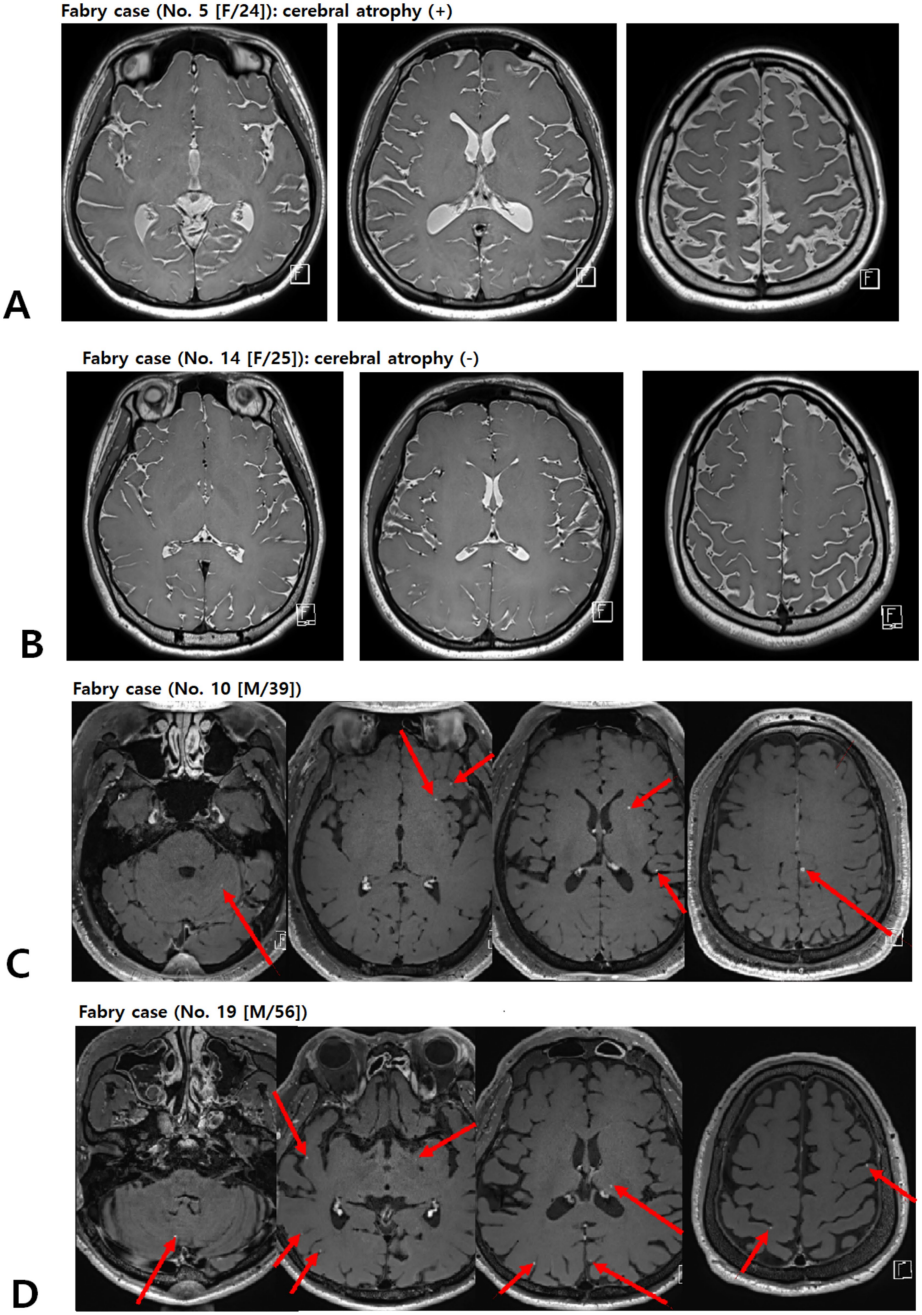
Representative picture of brain atrophy (A,B) and multiple enhancing lesions (C,D) in patients with FD. Contrast-enhanced T1-weighted images of patients with FD demonstrating multiple punctate/patchy parenchymal enhancing lesions (red arrows) (C and D). FD, Fabry disease.
